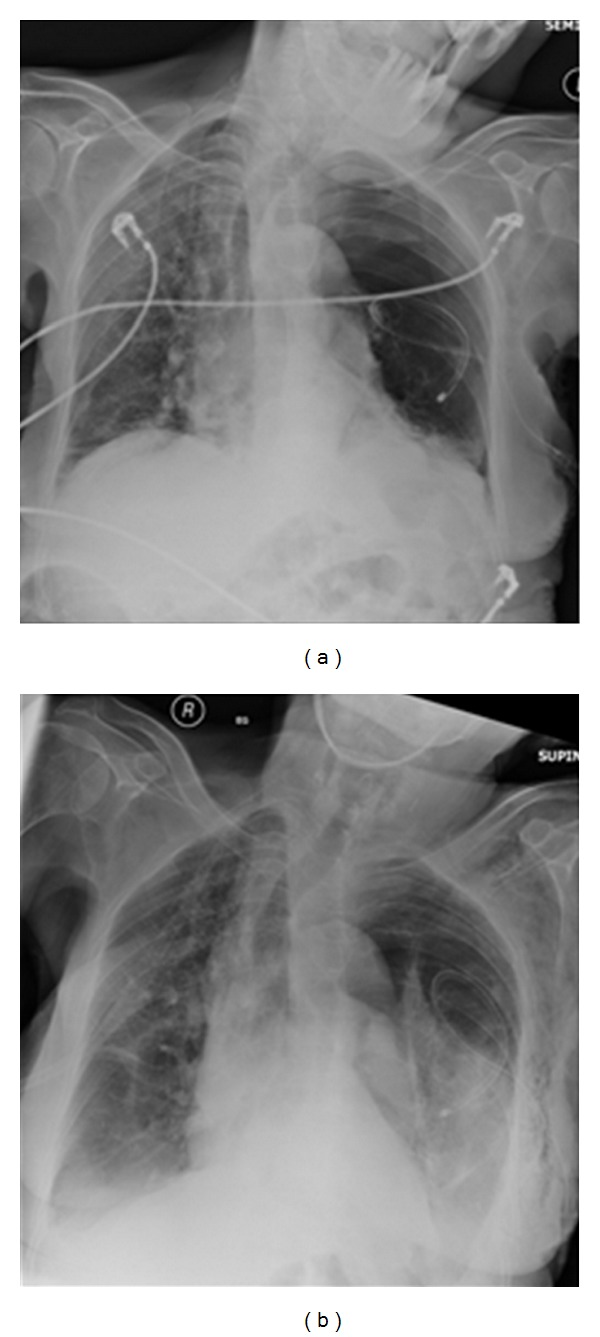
Two consecutive radiographs on the same patient as in Figure 24 showing a chest drain in situ at the left lung base. The patient's symptoms worsened following insertion of the chest drain. A repeat chest radiograph shows an accumulation of fluid at the left lung base.
